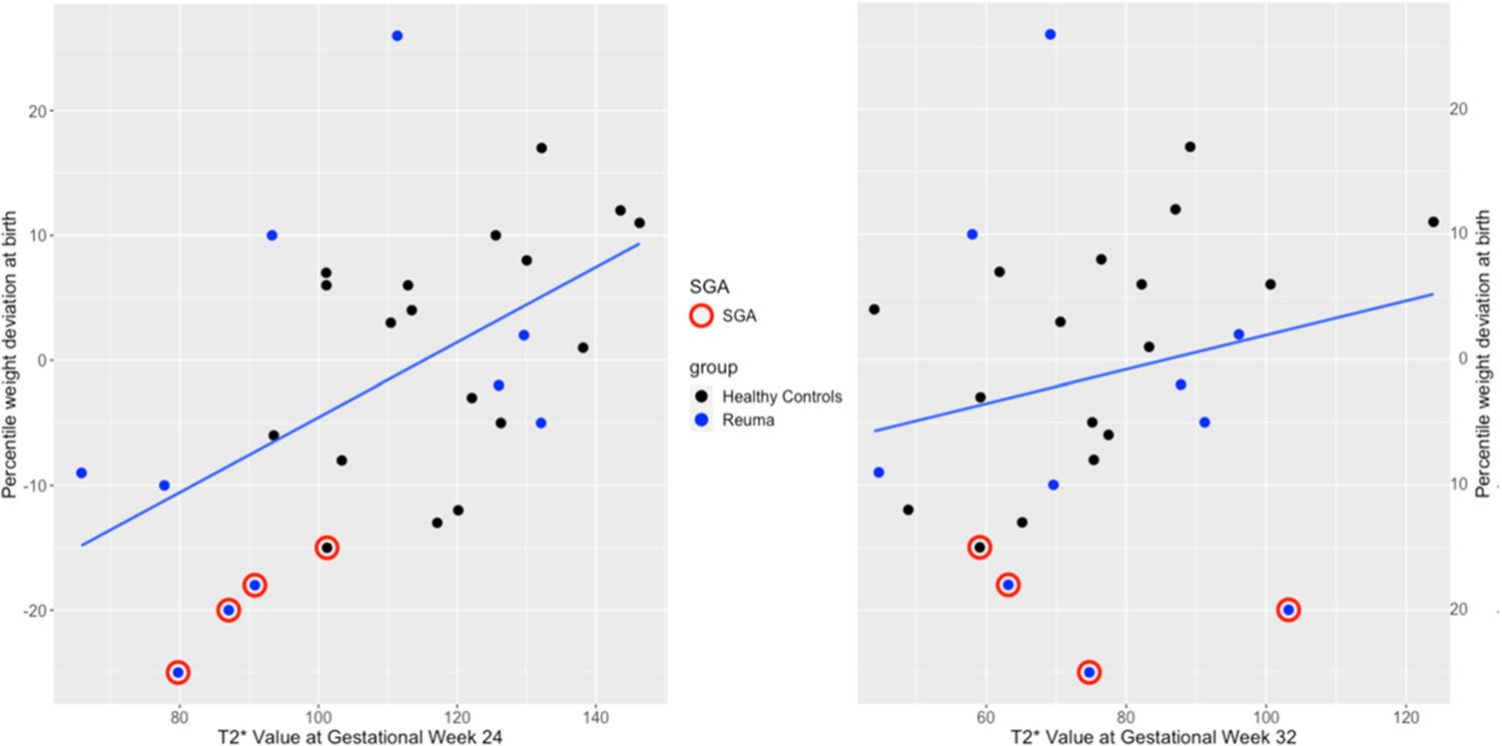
Correlation between ultrasound-assessed deviation from expected birthweight and T2*-weighted MRI at gestational week 24 (*r* = 0.52, *p* = 0.004) and 32 (*r* = 0.05, *p* = 0.29), respectively. Black = healthy controls; blue = chronic rheumatic disease; red circle = small for gestational age
